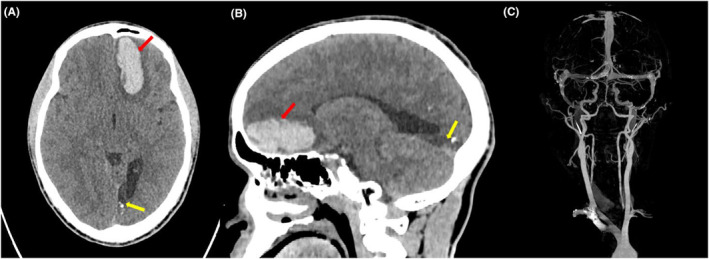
Plain CT head (A) Axial, and (B) Sagittal cuts showing an intra‐axial hematoma in the left frontal region (red arrows) with surrounding perifocal edema. A hypodense area in the left occipital region (yellow arrows) with calcifications and ex vacuo dilatation of the posterior horn of the left lateral ventricle due to the previous ischemic insult was also seen. (C) A CT intracranial angiogram is unremarkable.
